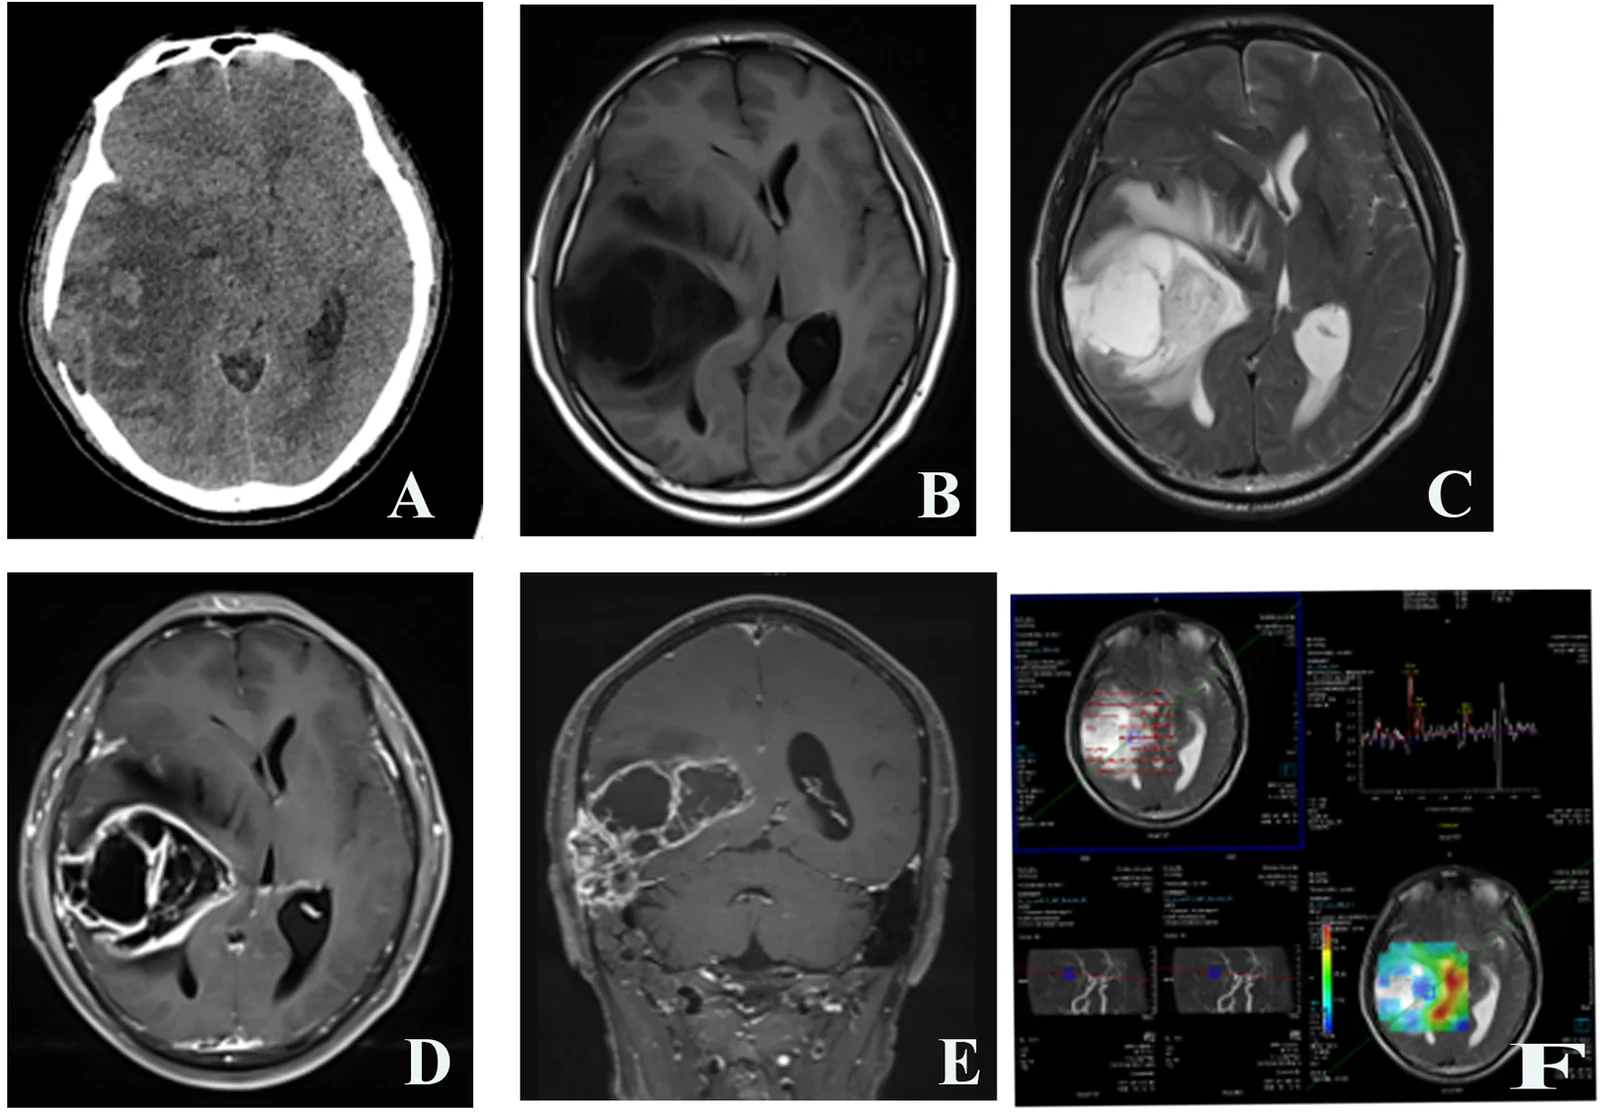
Preoperative CT, MRI and MRS of the patient. (A) Head CT demonstrated a mass lesion in the right temporal region, with adjacent osseous destruction and leftward midline shift. (B) Axial T1-weighted imaging (T1WI) showed the tumor as hypointense. (C) Axial T2-weighted imaging (T2WI) showed the tumor as hyperintense. (D) Axial contrast-enhanced T1WI revealed marked heterogeneous, ring-like enhancement of the tumor. (E) Coronal contrast-enhanced T1WI. (F) MRS showed a decreased NAA peak and an elevated Cho peak; the NAA/Cr ratio was 0.58, the Cho/Cr ratio was 2.46, the Cho/NAA ratio was approximately 4.21, and the lipid-lactate (LL) peak was elevated.
